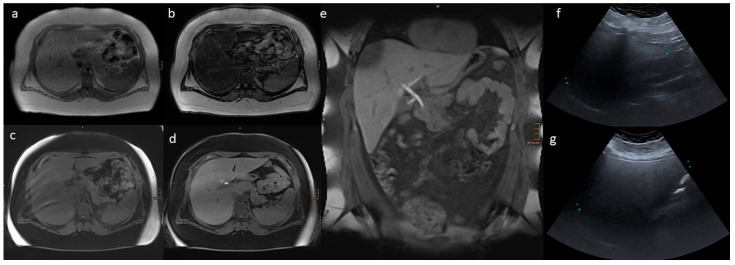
Liver MR and ultrasound images of a 14.6-year-old boy with a body mass index of 56.3 kg/m^2^: (a) In-phase; (b) opposed phase; (c) non-contrast T1 VIBE fat saturated; (d,e) transitional phase of Gd-EOB-DTPA-enhanced T1 VIBE fat saturated sequences; and (f) AAL and (g) MCL liver ultrasound images. In the ultrasound, a severe steatosis hepatis was estimated according to the echogenicity of the liver parenchyma. In the MRI, the liver volume was 3401 mL, and the hepatic fat fraction was 38.4% based on severe steatosis hepatis, and 0.36 was the relative enhancement.
